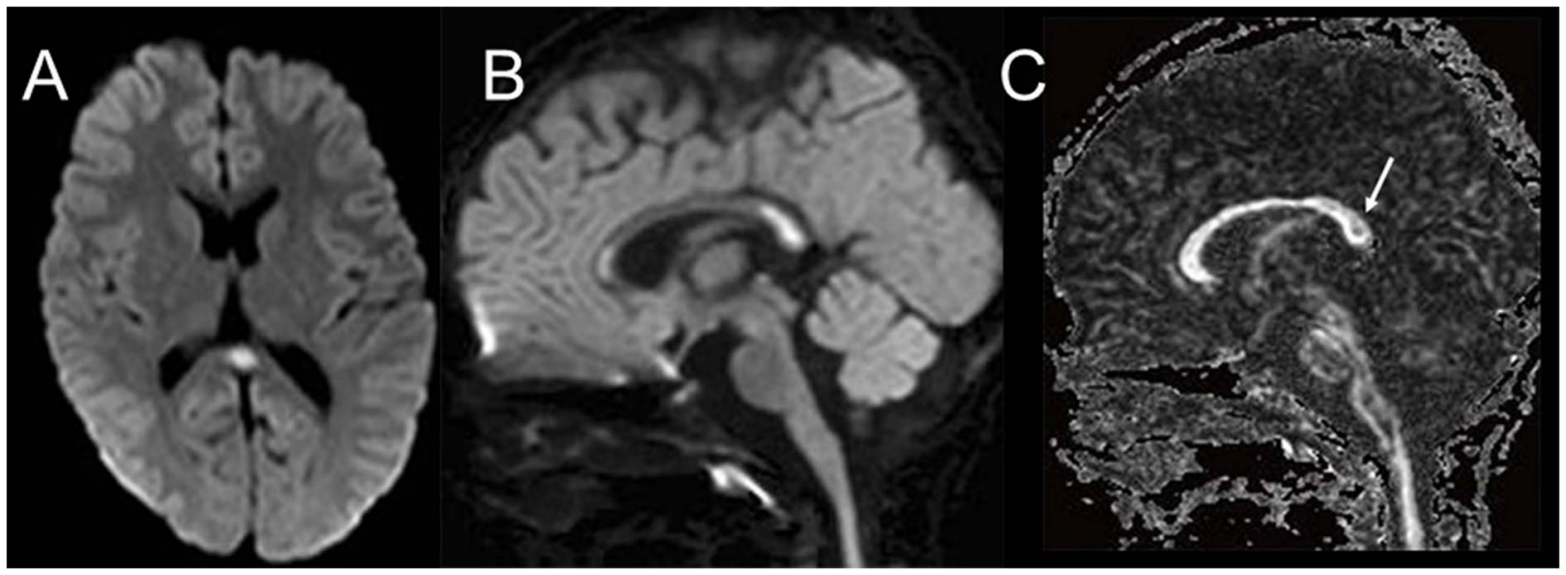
MRI in a teenager with MERS type 1. DWI (A,B) show a high-signal lesion in the splenium and genu of the corpus callosum, with reduced diffusion. The FA map shows reduced FA (C), all of which disappeared on the follow-up MRI. MERS, clinically mild encephalitis/encephalopathy with reversible splenial lesions; DWI, diffusion-weighted imaging; FA, fractional anisotropy; MRI, magnetic resonance imaging.
